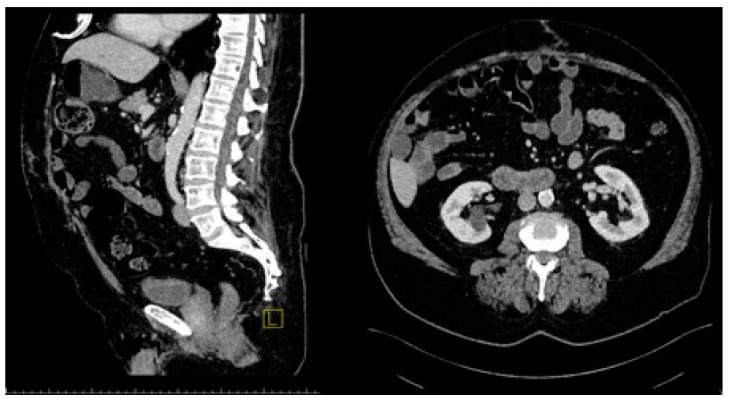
Computed tomography (CT) images, with sagittal and vertical views showing the mesh and overlapped fascia without bulging. (L on the image: left side).
